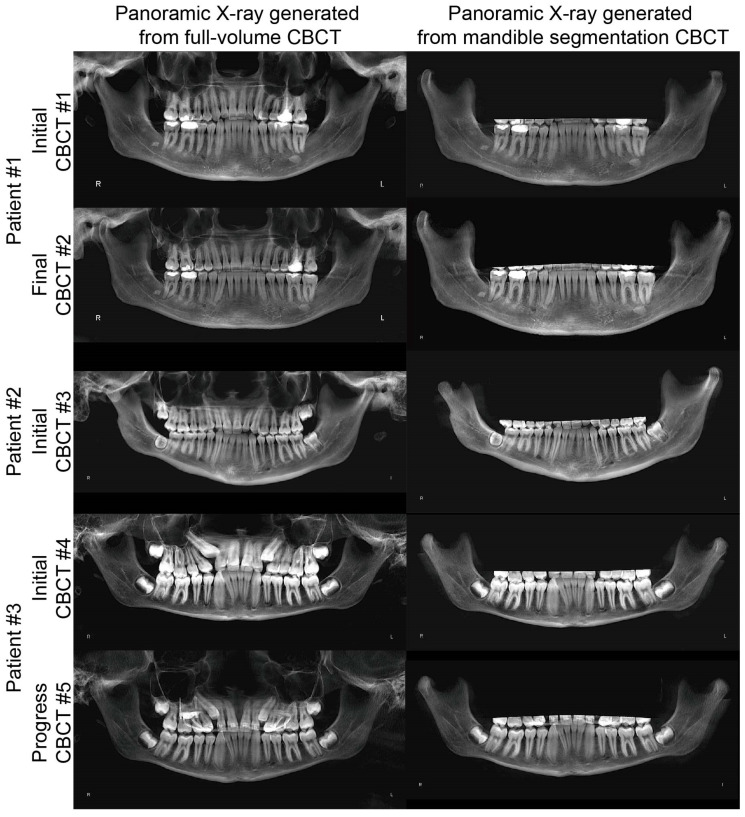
Comparison of the panoramic radiographs generated from the full-volume CBCT and those from the segmented mandible CBCT.
